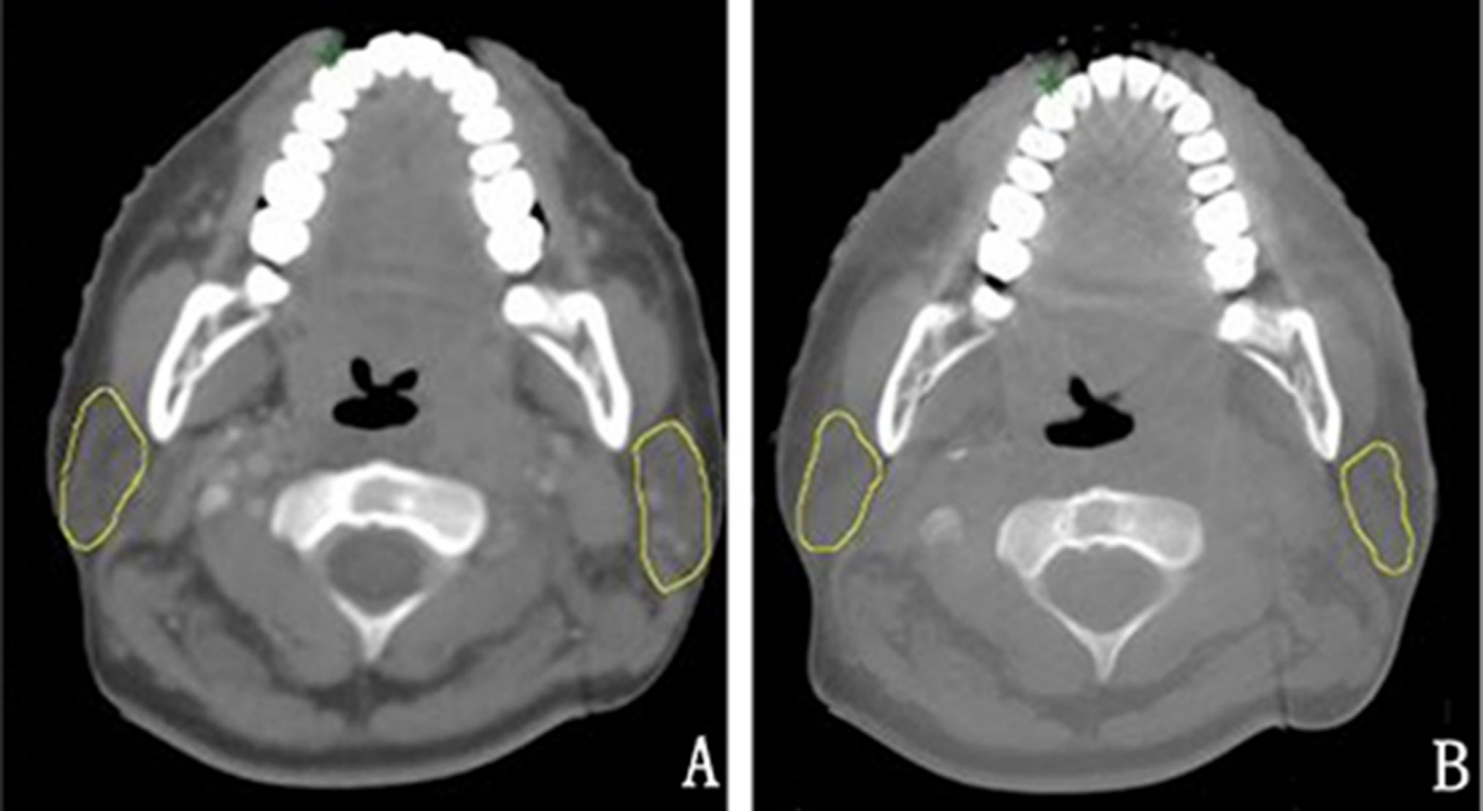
The contrast of the nasopharynx in the transverse section between the planning CT image and CBCT image before the first radiation from one patient A. the planning CT B. CBCT before the first radiation.
